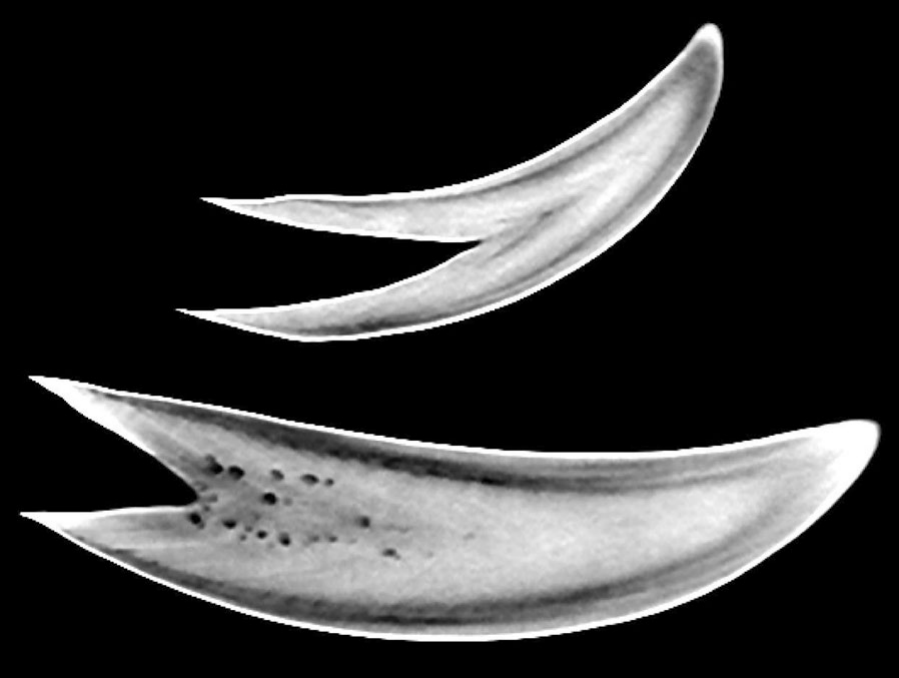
CT images of both teeth (tooth A and B) using Somatom Definition Flash and the scan- and reconstruction parameters in the CT method details. Coarse inner structures such as *small inner holes* and different densities of enamel and marrow are clearly visualized. Parts of the V-shaped structures can be seen
